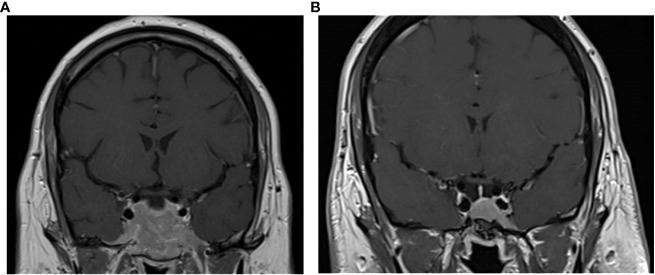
Coronal T1-weighted MRI images with contrast enhancement of the pituitary tumor at presentation in 2008 (A) and at last follow-up in 2022 (B).
